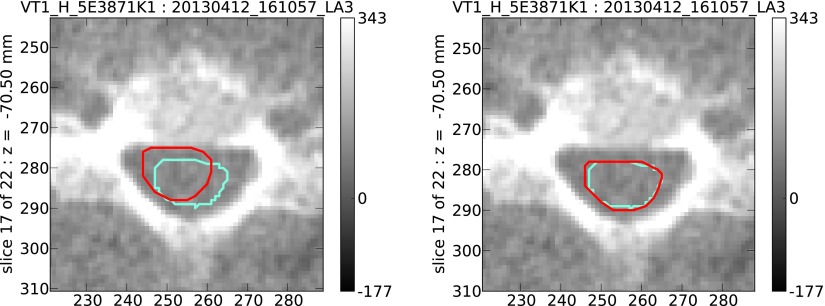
Spinal cord contours on a single MVCT scan slice; manual contours (cyan) and contours propagated form the patient’s kVCT scan (red). In this example, agreement between the manual contour and a propagated contour using an affine plus B-spline transform (right image, CI 0.88) is significantly better than agreement between the manual contour and the propagated contour using radiographer couch shifts (left image, CI 0.49).
